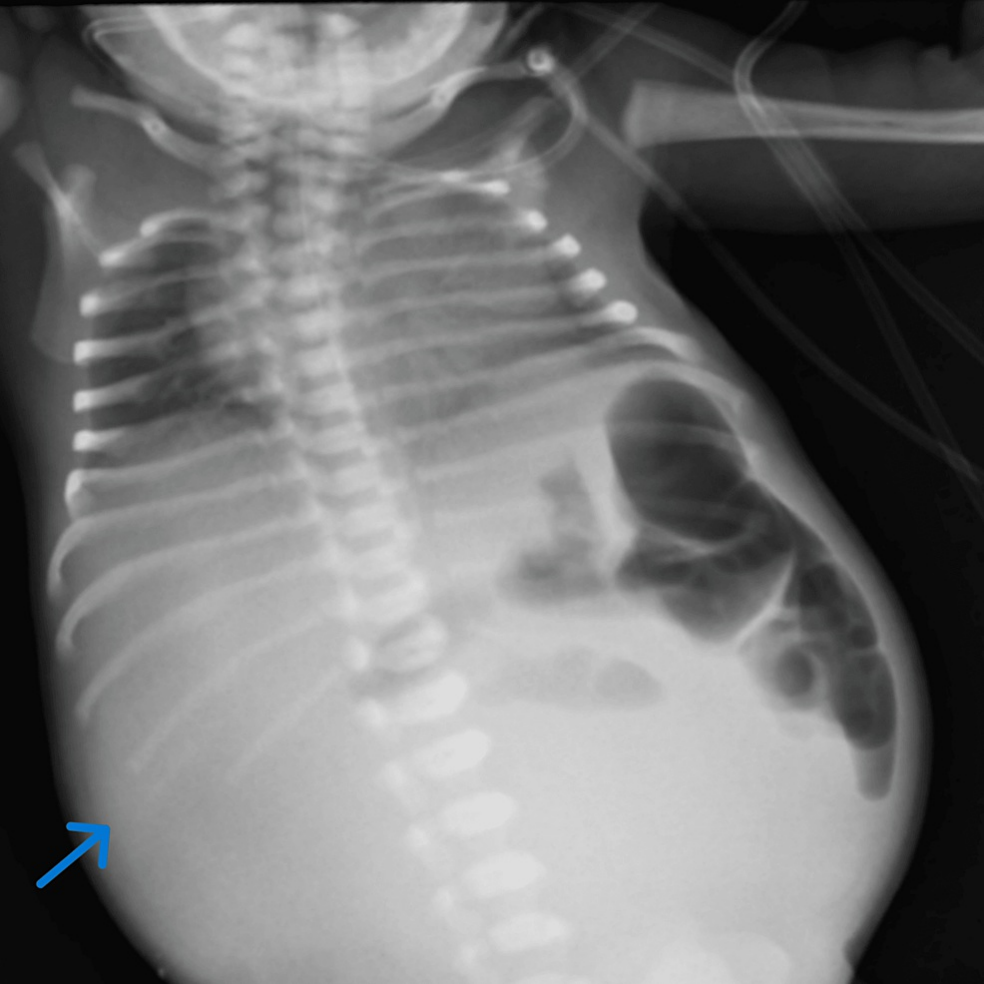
X-ray of a neonate with a meconium pseudocyst resulting from bowel perforation.
